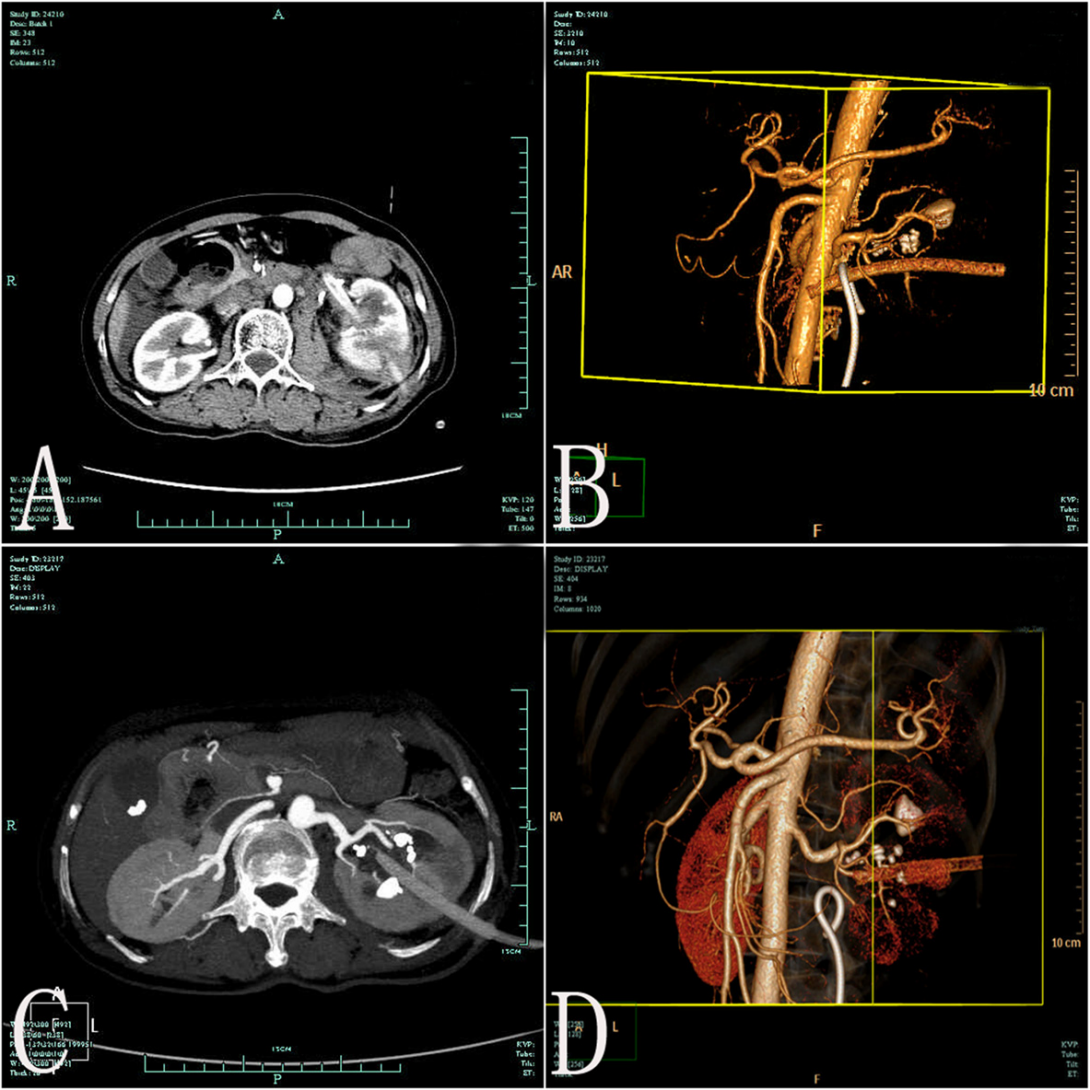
Axial contrast enhanced CT and volume rendering image of CT angiography. A-B) Axial contrast enhanced CT and volume rendering image of CT angiography demonstrate percutaneous nephrostomy drainage tube inside the left renal vein; C-D) Axial contrast enhanced CT and volume rendering image of CT angiography reveal percutaneous nephrostomy drainage tube inside the left renal pelvis after the percutaneous nephrostomy drainage tube was withdrawn in stages.
